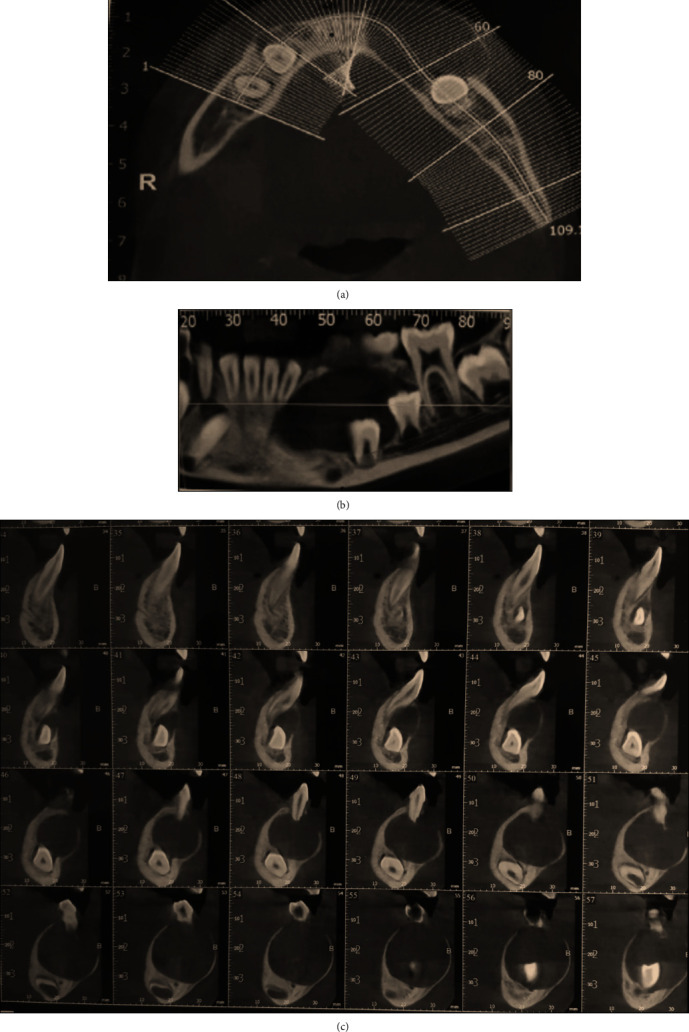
(a) Axial view of the CBCT showing the expanded buccal bone. (b) Panoramic reconstruction revealed a well-limited, unilocular radiolucent image apical to the deciduous teeth with an extension to the mesial of the tooth #36, and the crowns of the unerupted teeth #33, #34, and #35 were pushed towards the basilar mandibular bone. (c) Para-axial cuts showed apical displacement of the teeth buds towards the mental nerve in proximity and an expansion of the buccal and lingual bone plates.
